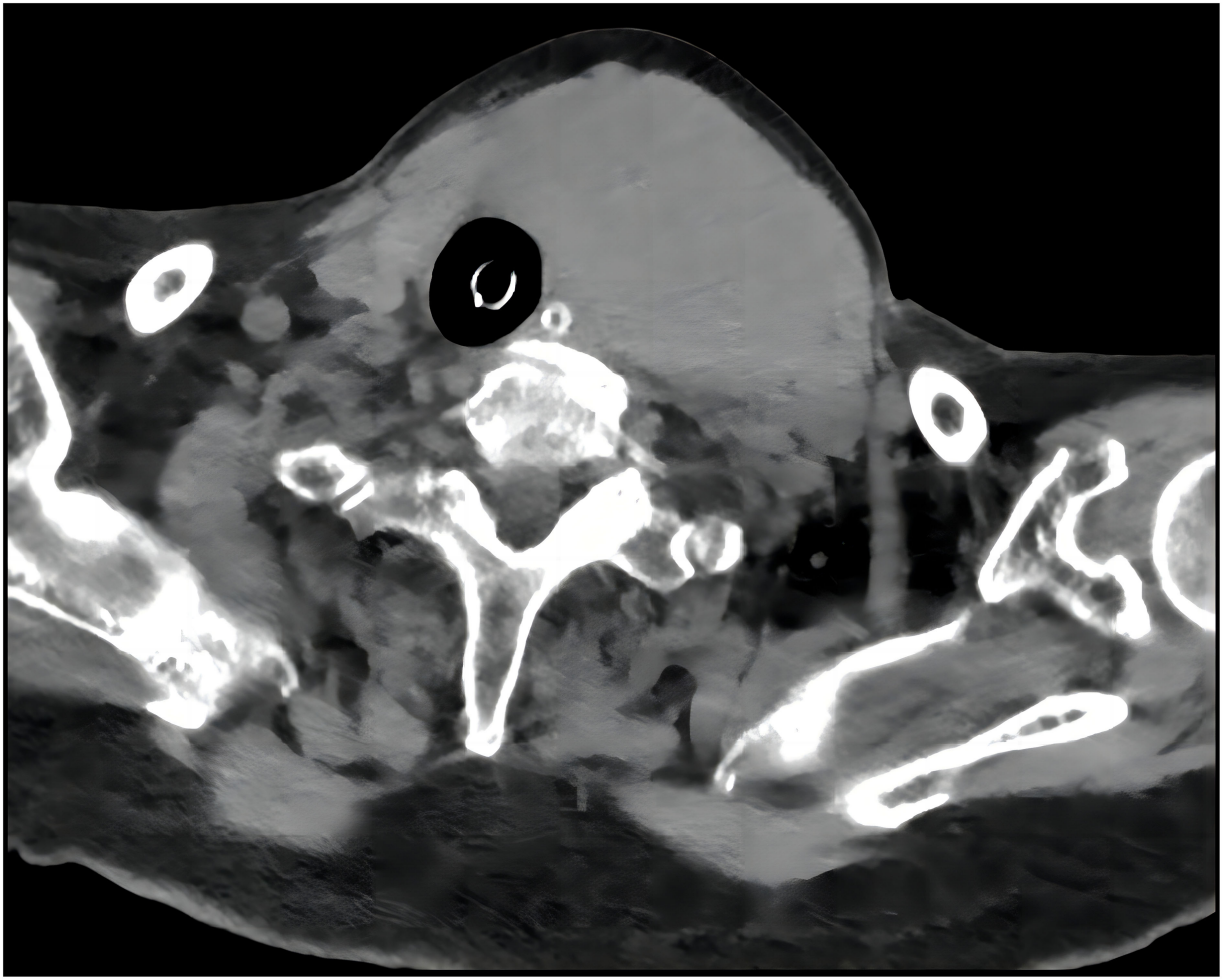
A CT of the neck revealed a solid lesion in the left thyroid lobe causing tracheal compression and deviation with endotracheal intubation.
